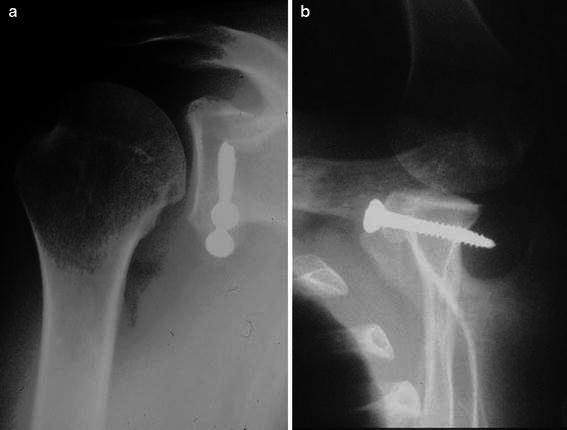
Postoperative X-ray control: AP view (a) and Bernageau view (b), showing that the graft has perfect positioning, with no overhang into the joint
